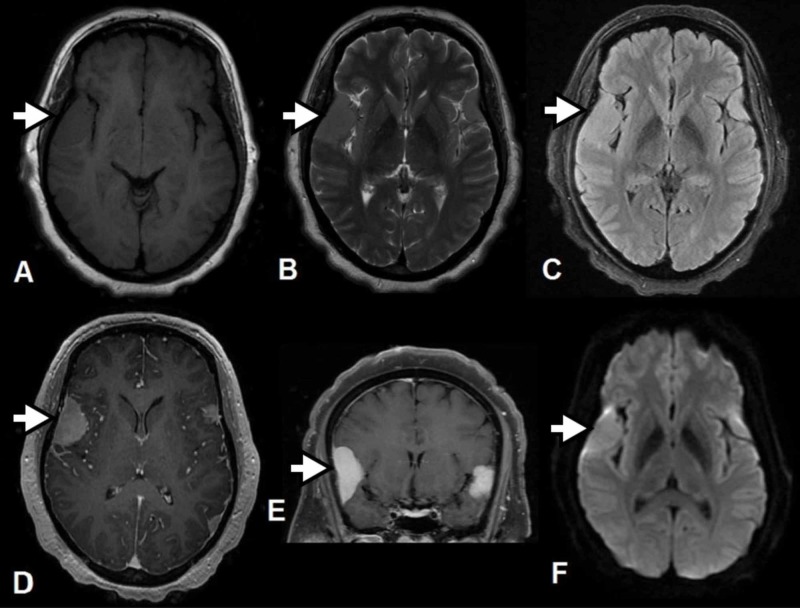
Magnetic Resonance Imaging With and Without Contrast Images Axial T1 (A), axial T2 (B), axial FLAIR (C), axial and coronal T1 post-contrast (D&E), and axial DWI (F) sequences show dural based extra-axial supratentorial lesions along the bilateral paramidline frontal and right parietal lobes at the vertex, left frontal lobe near the vertex, bilateral posterior parietal lobes, and right greater than left frontal-temporal lobes. These lesions were homogeneously enhancing, partially diffusion restricting, and without vasogenic edema in the adjacent parenchyma.
